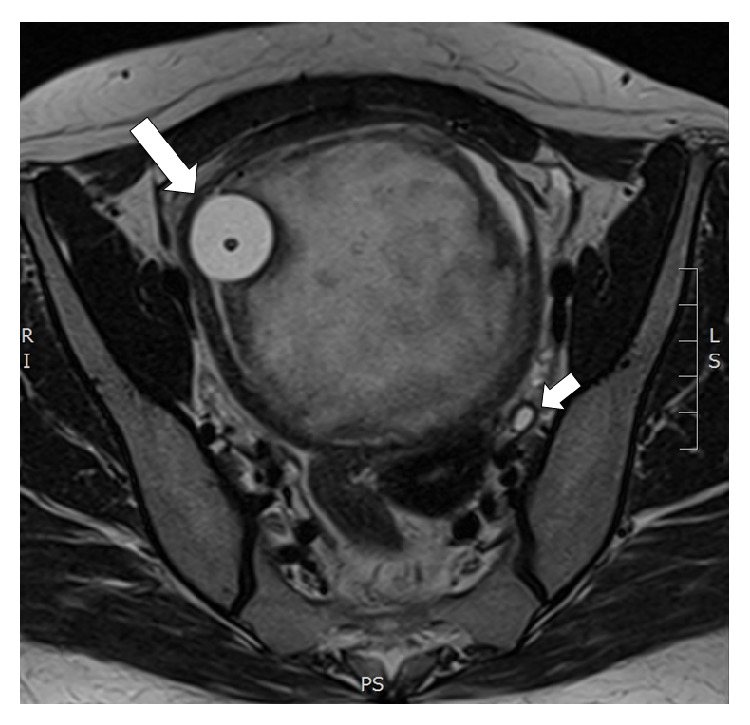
Axial section of T2 weighted MRI pelvis demonstrating solid mass within the bladder displacing the catheter balloon (large arrow) and causing bilateral hydronephrosis (small arrows).
